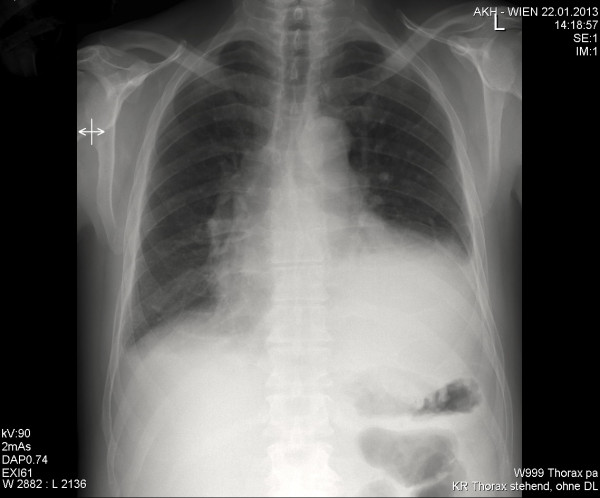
Preoperative chest X-ray, showing cardiomegaly suspicious of true left ventricular aneurysm complicating myocardial infarction. Frontal view. Abnormal evagination of left cardiac border is typical for an aneurysm involving the anterolateral and/or apical segment of the left ventricle. The aneurysm bulges out and stretches to the thoracic wall. There is associated pulmonary congestion due to heart failure.
